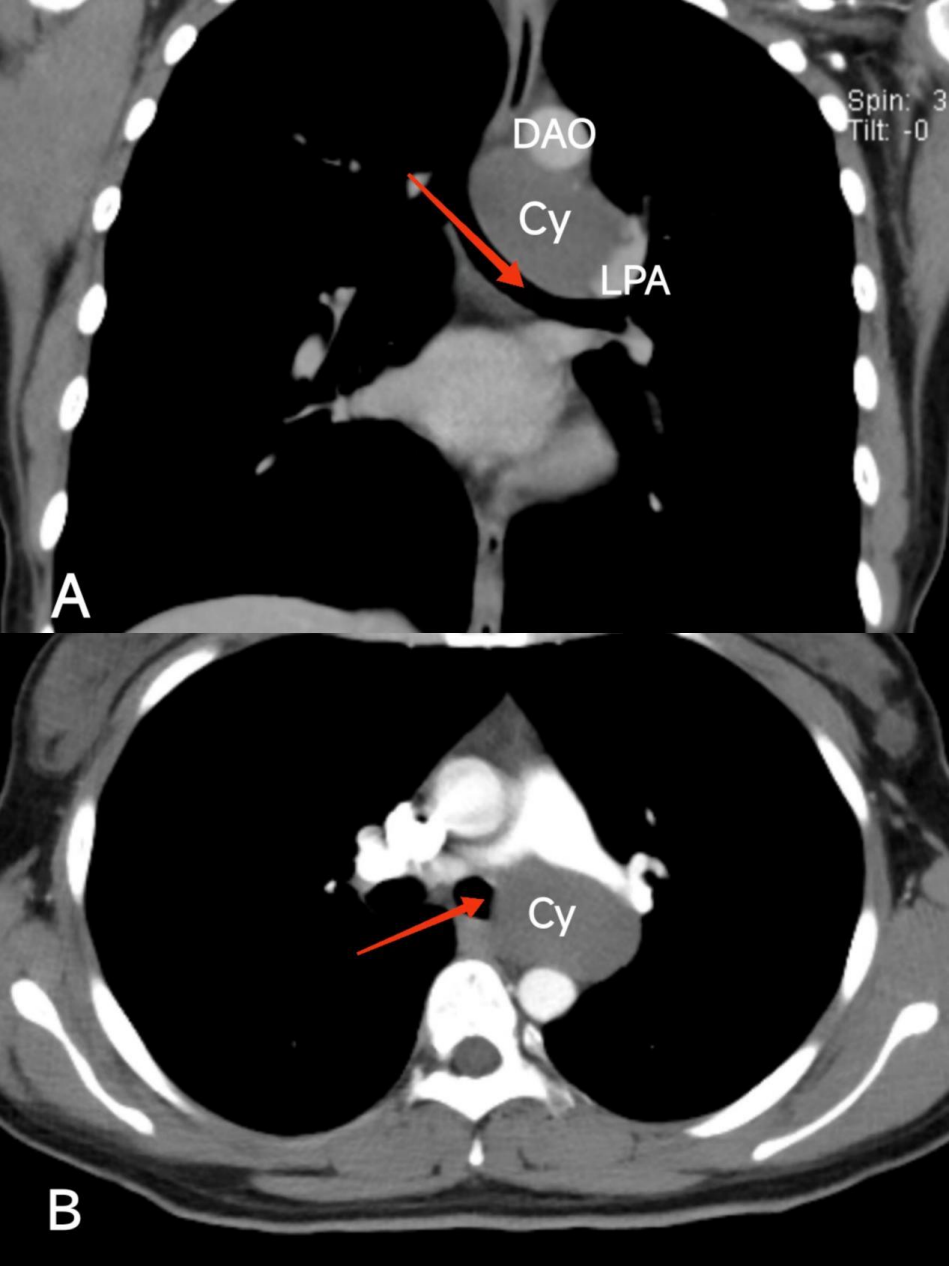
Chest axial and coronal CT scans showing the adjacent relationship and characteristics of the lung cyst. (Cy: cyst, LPA: left pulmonary artery, DAO: descending aorta, red arrow: left main bronchus).
